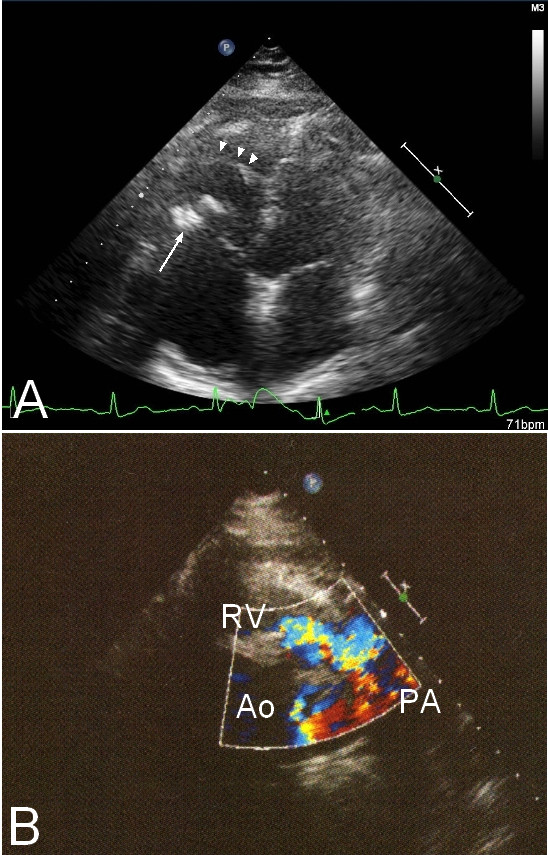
Transthoracic echocardiography. (A) Apical four chamber view shows anomalous muscle bundle (arrowheads) and calcification of tricuspid valve (arrow). (B) Color Doppler study from parasternal short axis view shows high velocity flow signal in RVOT.
